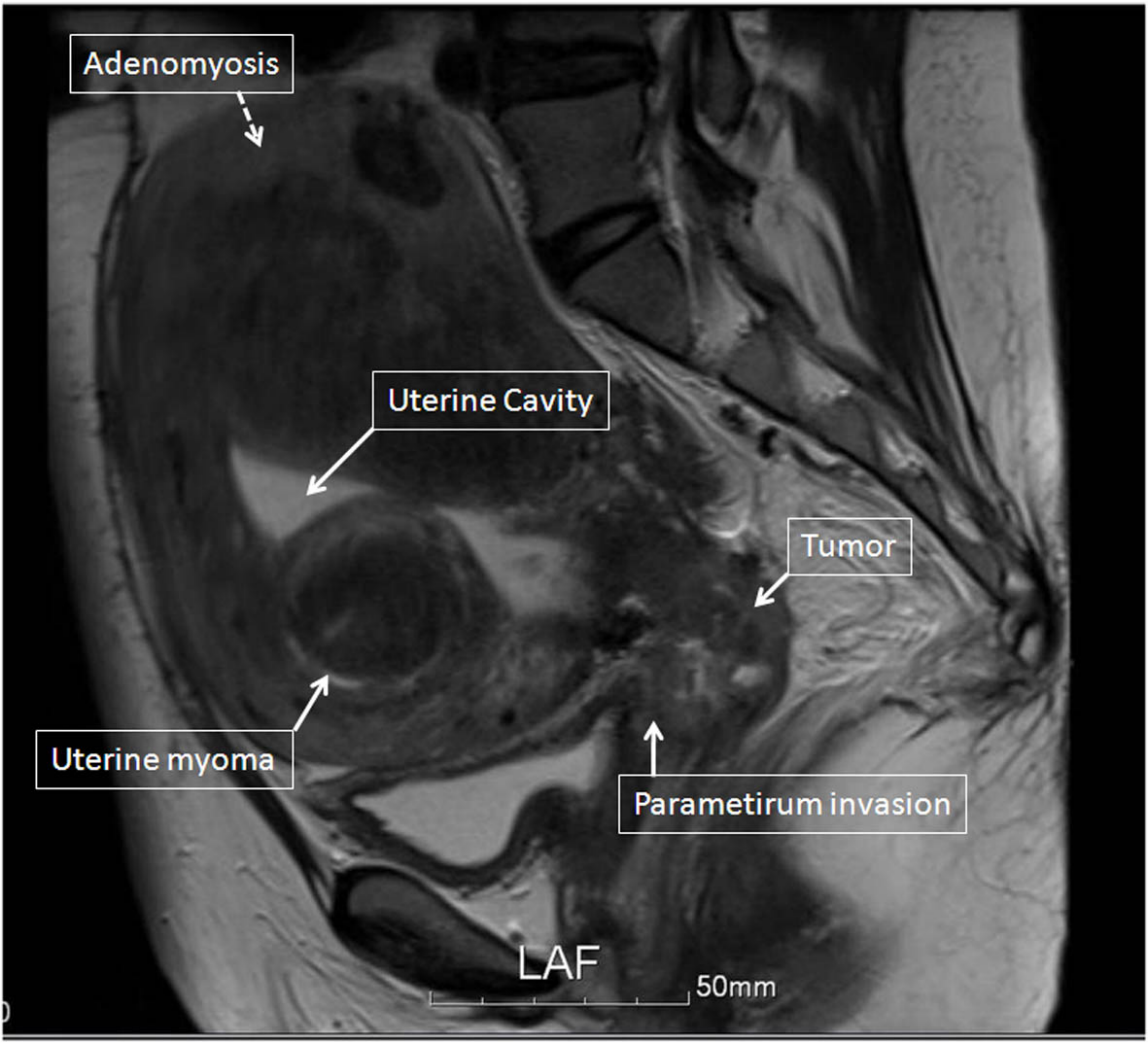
MR image of the pelvis before treatment shows direct invasion of the cervical tumor into the right parametrium, bilateral hydronephrosis, and multiple uterine myomas. The solid arrows indicate the parametrium invasion, the uterine myomas, the tumor, and the uterine cavity. The dotted arrow indicates adenomyosis.
